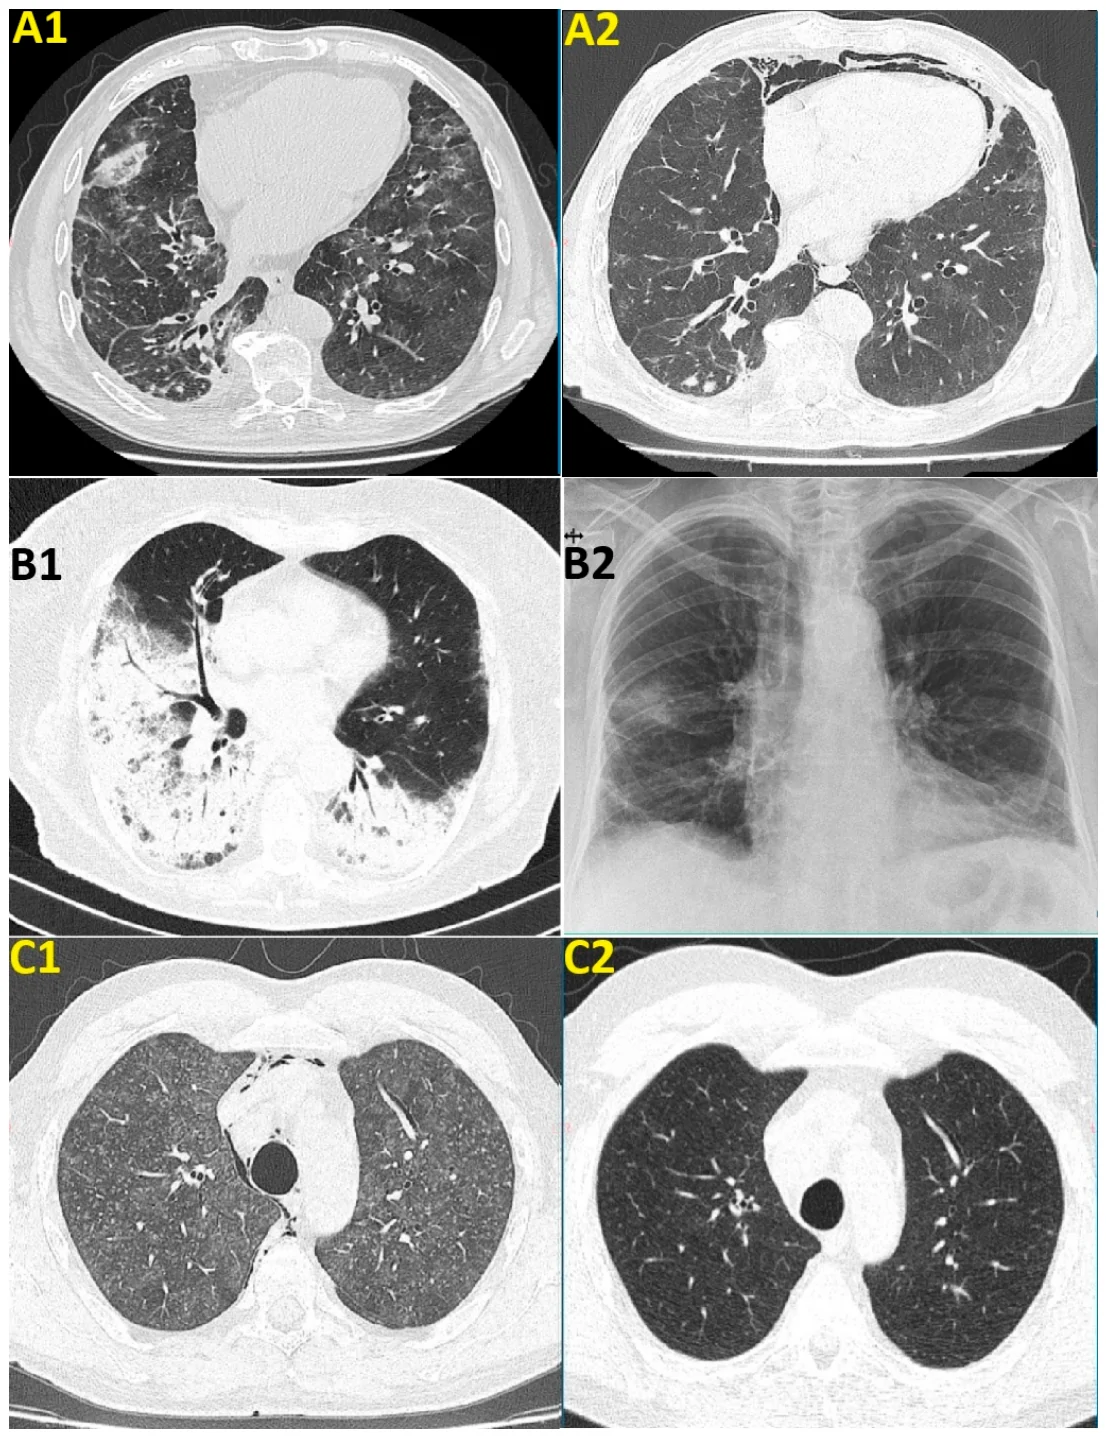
Radiologic patterns of oncologic treatment-induced pneumonitis; (A1) case 10 treated with nivolumab—nonspecific interstitial pneumonia (NSIP)-like pattern on thoracic CT; (A2) case 10 after 1 month of corticotherapy, with pneumomediastinum; (B1) case 6 treated with pembrolizumab—organizing pneumonia (OP) pattern on thoracic CT; (B2) case 6’s chest X-ray after 1 month of corticotherapy; (C1) case 9 treated with ipilimumab/nivolumab—hypersensitivity pneumonitis (HP) pattern on thoracic CT; (C2) case 9 after 1 month of corticotherapy. (See Table 5 and Figure 3 for corticotherapy regimens.).
